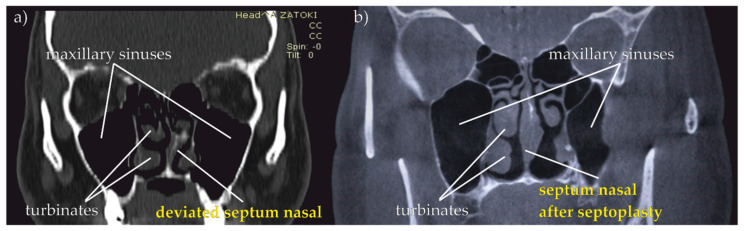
Computed Tomography scan of a patient with deviated septum nasal (a) before surgery; (b) after surgical intervention.
